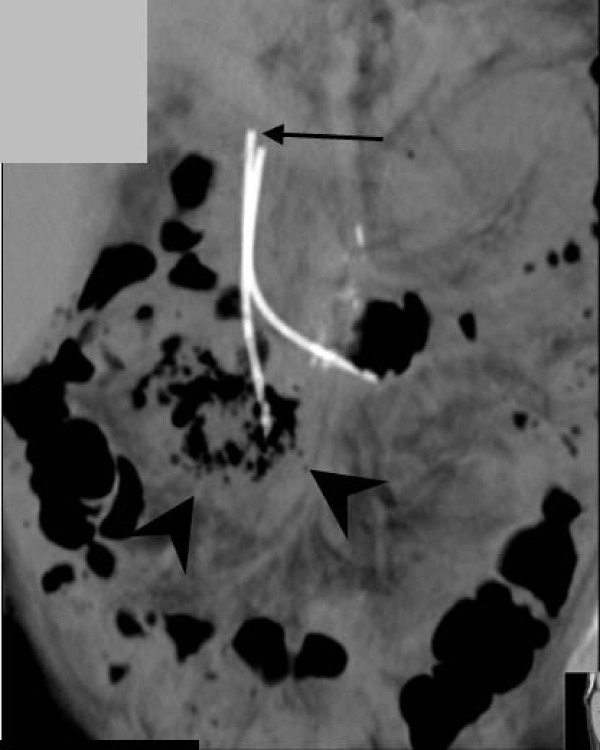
Coronal reformat computed tomography image. One of the stents has migrated (white arrow) and its tip is lying in a large, gas-filled retroperitoneal abscess (black arrowheads).
